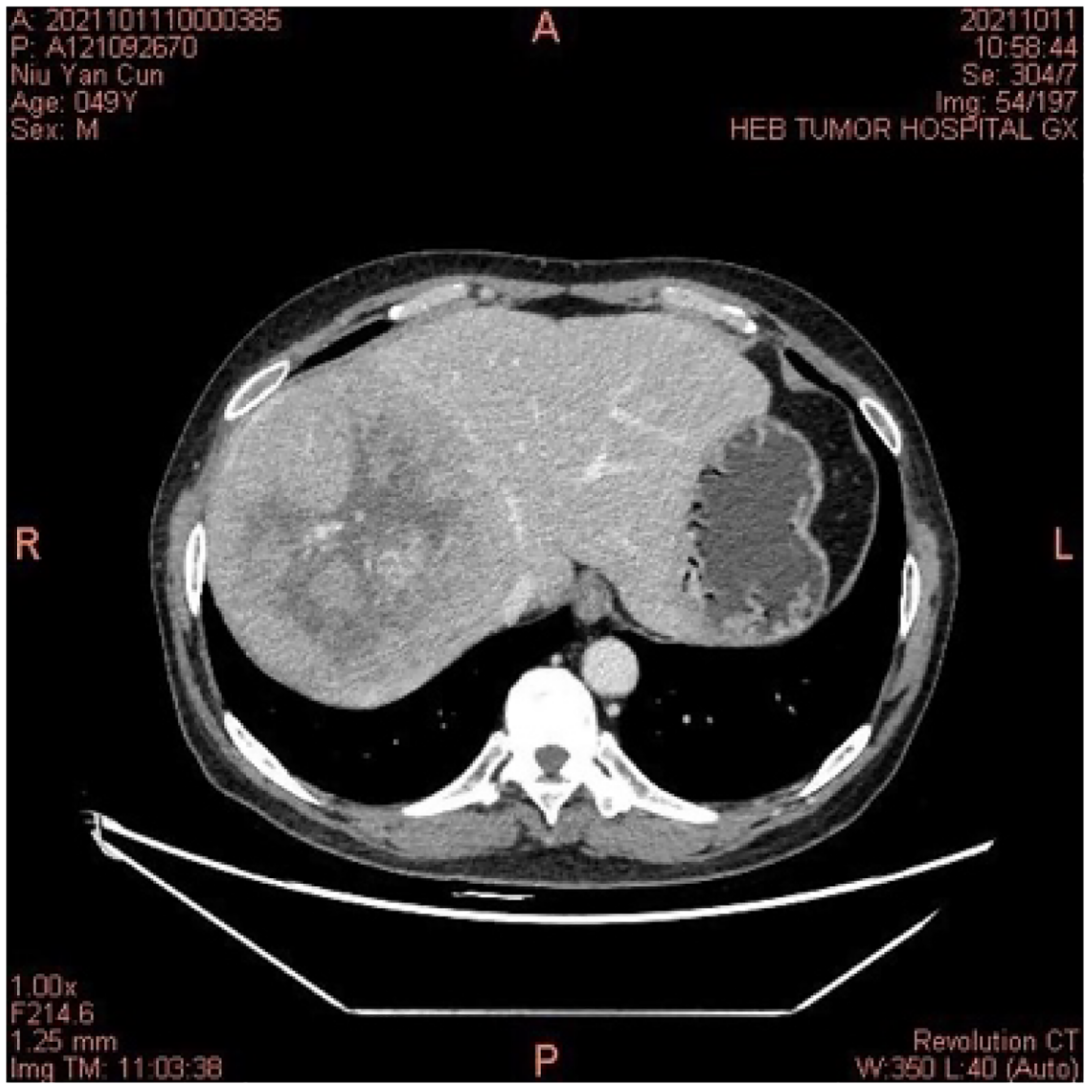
In October 2021, the computed tomography of the abdomen indicated a space-occupying lesion in the right lobe of the liver, and liver cancer was considered.
